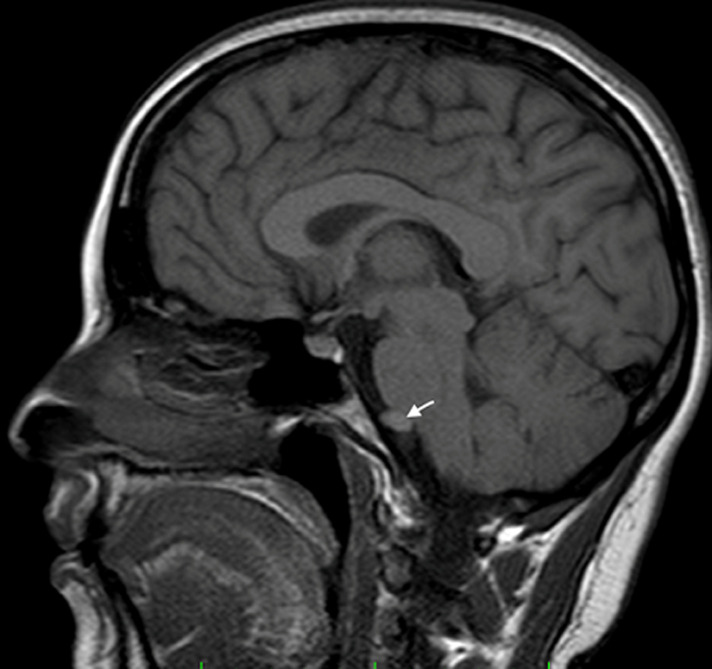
sagittal T1 MRI showing the isointense cystic extra axial lesion anterior to the brainstem
